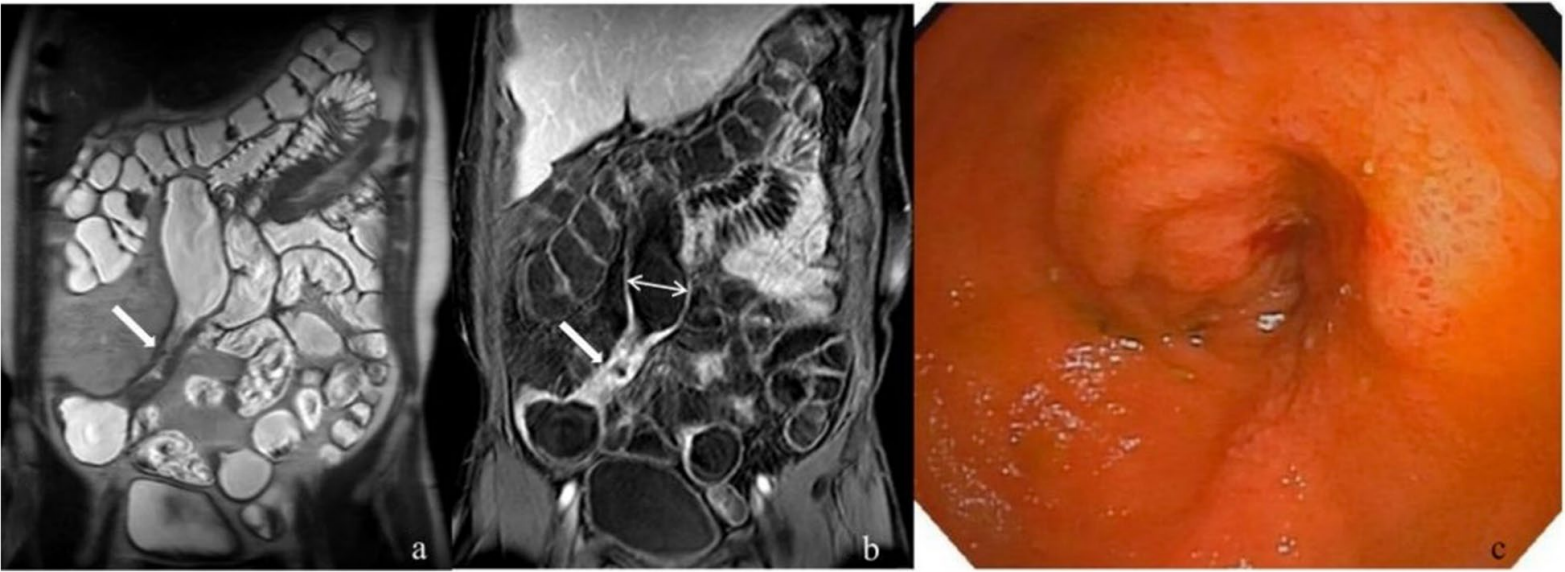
A 37-year-old man with Crohn’s disease. a Coronal T2-weighted imaging before endoscopy: marked thickening of the intestinal wall, narrowing of the intestinal cavity, and normal signals (*white arrow*). b Coronal enhanced T1-weighted imaging with dilation of the upper intestinal cavity (*double-ended white arrow*) and moderate and homogenous enhancement of the intestinal wall (*white arrow*). c The endoscopy showed a narrow ileum, but the endoscopy could not pass
